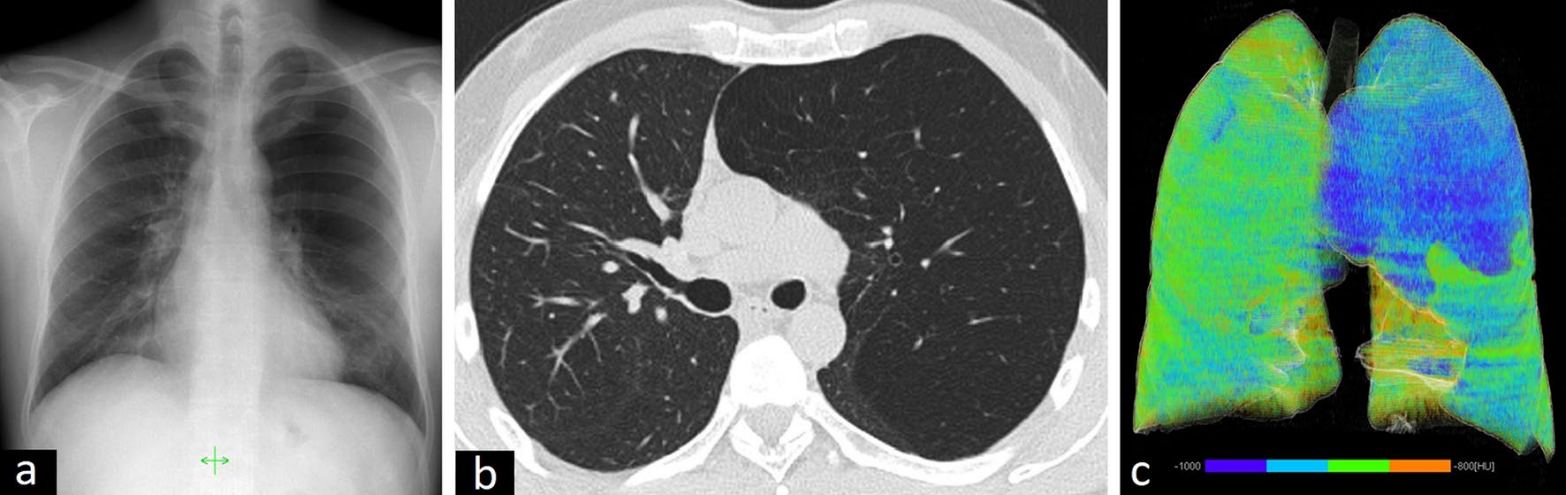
Radiological features of congenital lobar hyperinflation in a 42-year-old male presenting with frequent respiratory infections. Chest X-ray shows hyperlucency of the left lung (a). Axial CT image (b) and quantitative 3D reconstruction image (c) demonstrate lobar hyperinflation (c, blue area) and shift to the contralateral side
